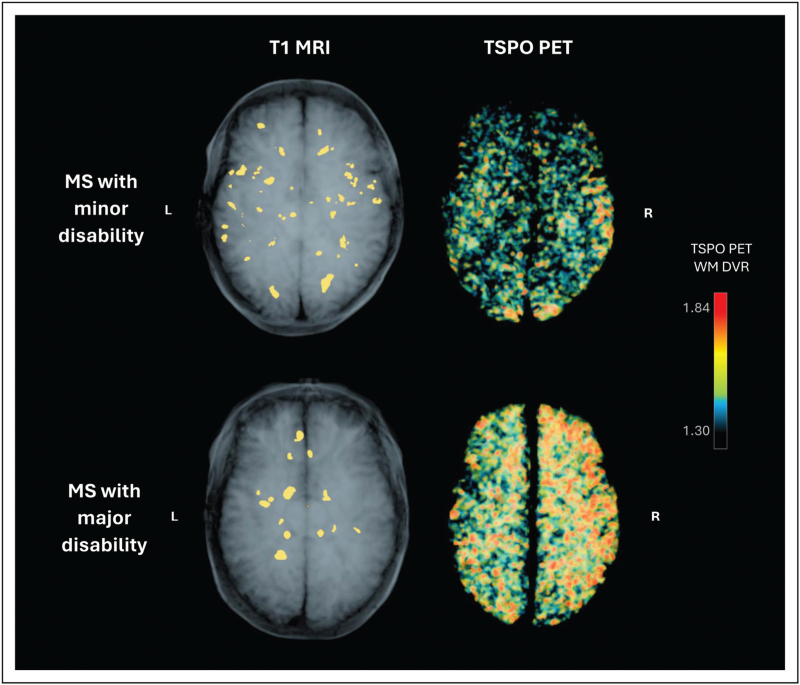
Lesion locations and areas of high microglial activation in the white matter of female MS patients with either minor disability (EDSS 1.0, disease duration 9.6 years) or major disability (EDSS 6.0, disease duration 7.6 years). Microglial activation, as measured by TSPO distribution volume ratio (DVR), extends beyond focal MRI lesions and reveals smouldering inflammatory activity invisible to MRI particularly in the patient with major disability. Volumetric MRI and white matter (WM) TSPO PET images are rendered with transparency to visualize lesion locations and TSPO availability throughout the WM volume. T1 MRI image is overlayed with MS lesion locations.
